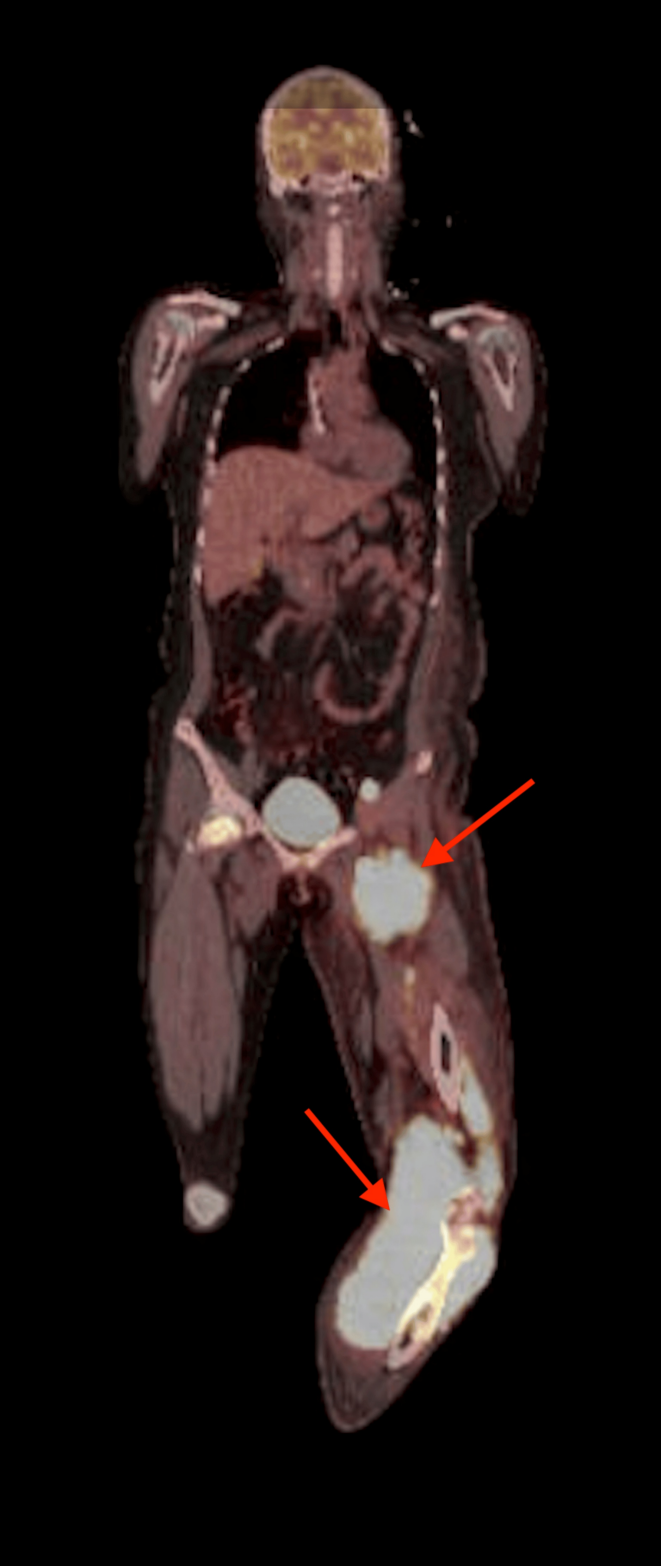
PET/CT showing intense radiotracer uptake within a left anterior thigh soft-tissue density mass measuring 7.5 × 9.4 cm and a left knee multicompartment soft-tissue density mass measuring 13.6 × 10.2 cm (arrows). PET/CT, positron emission tomography/computed tomography
